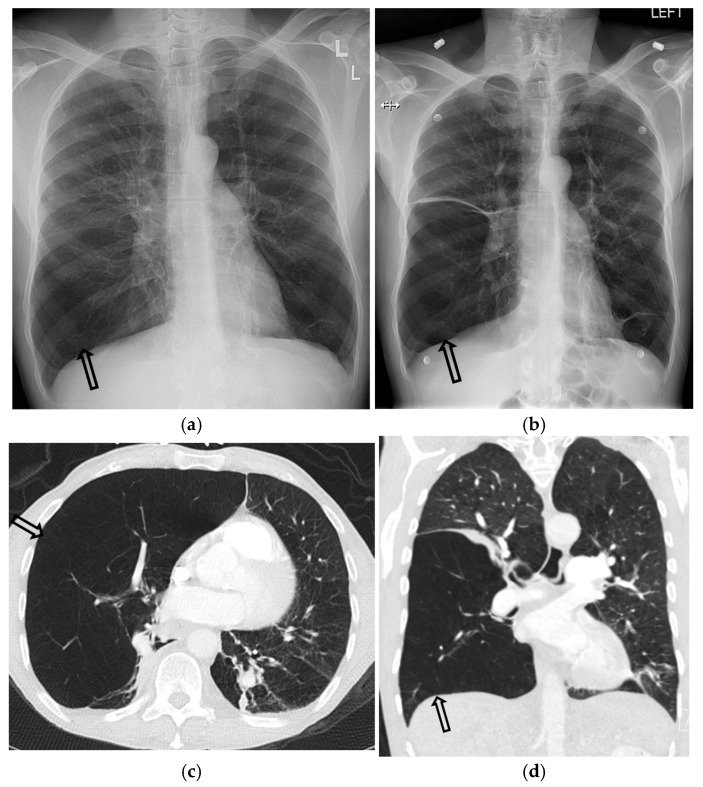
63-year-old male former smoker with a history of cocaine and intravenous ritalin use presents with chest pain. Initial frontal chest radiograph (a) and subsequent frontal chest radiograph performed 10 years later (b) show basilar-predominant hyperlucency of lungs (open black arrows). Axial and coronal CT images in lung window (c,d) show right-lower-lobe predominant panlobular emphysema (open black arrows), consistent with ritalin lung.
